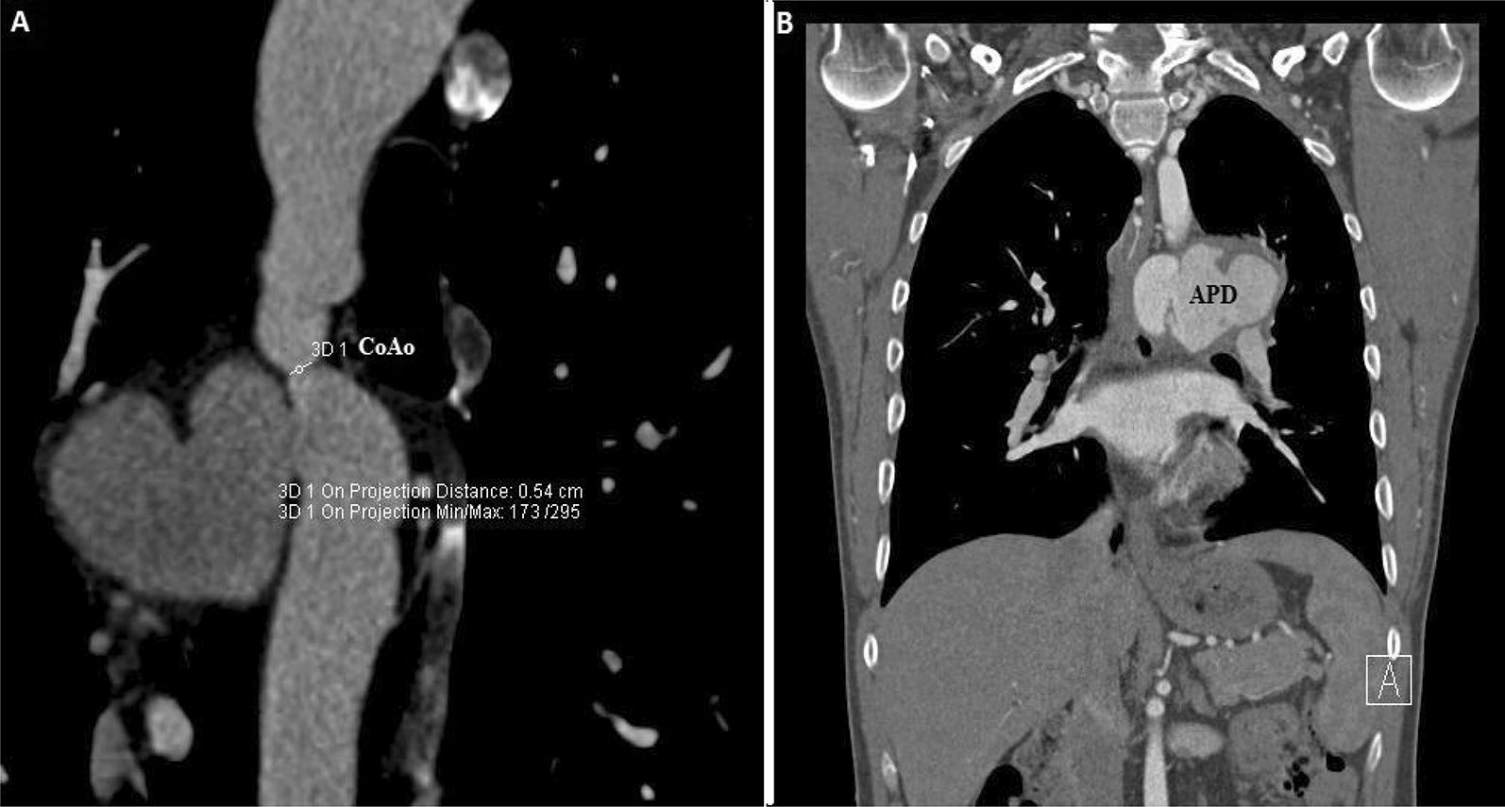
2D Computed tomography angiography scan shows a severe aortic coarctation and a giant pseudo-aneurysm at coarctation site (*CoAo* aortic coarctation, *APD* aortic pseudo-aneurysm)
